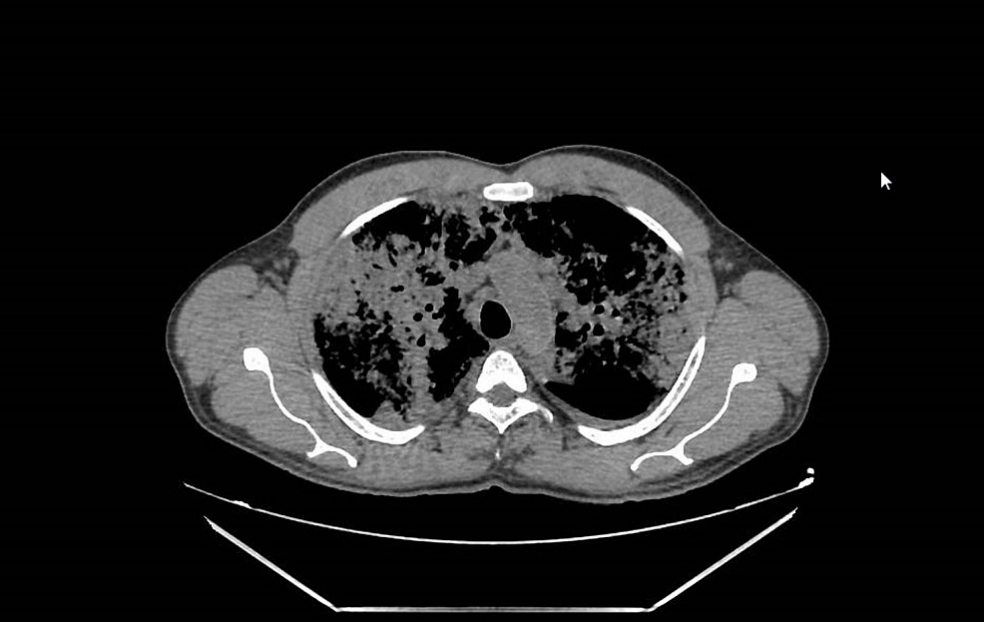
Chest CT Chest CT showing thickened bronchial walls, areas of ground glass in the upper lobes, and peripheral predominance, assuming the appearance of consolidation in some areas. CT, Computed tomography.
